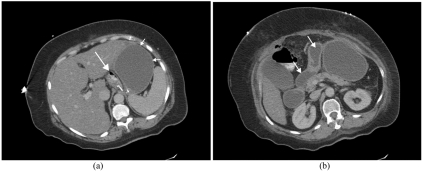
CT examination of the upper abdomen with IV and oral contrast. Acute dilatation of the distal gastric remnant in a patient presenting with severe upper abdominal pain and electrolyte imbalance two weeks after bypass surgery. (a) Dilated distal defunctioned stomach (short arrows), collapsed gastric pouch (long arrow) which contains some oral contrast. (b) Image inferior to (a), dilated duodenum (white arrow) and proximal jejunum (white arrow). This settled with conservative management.
